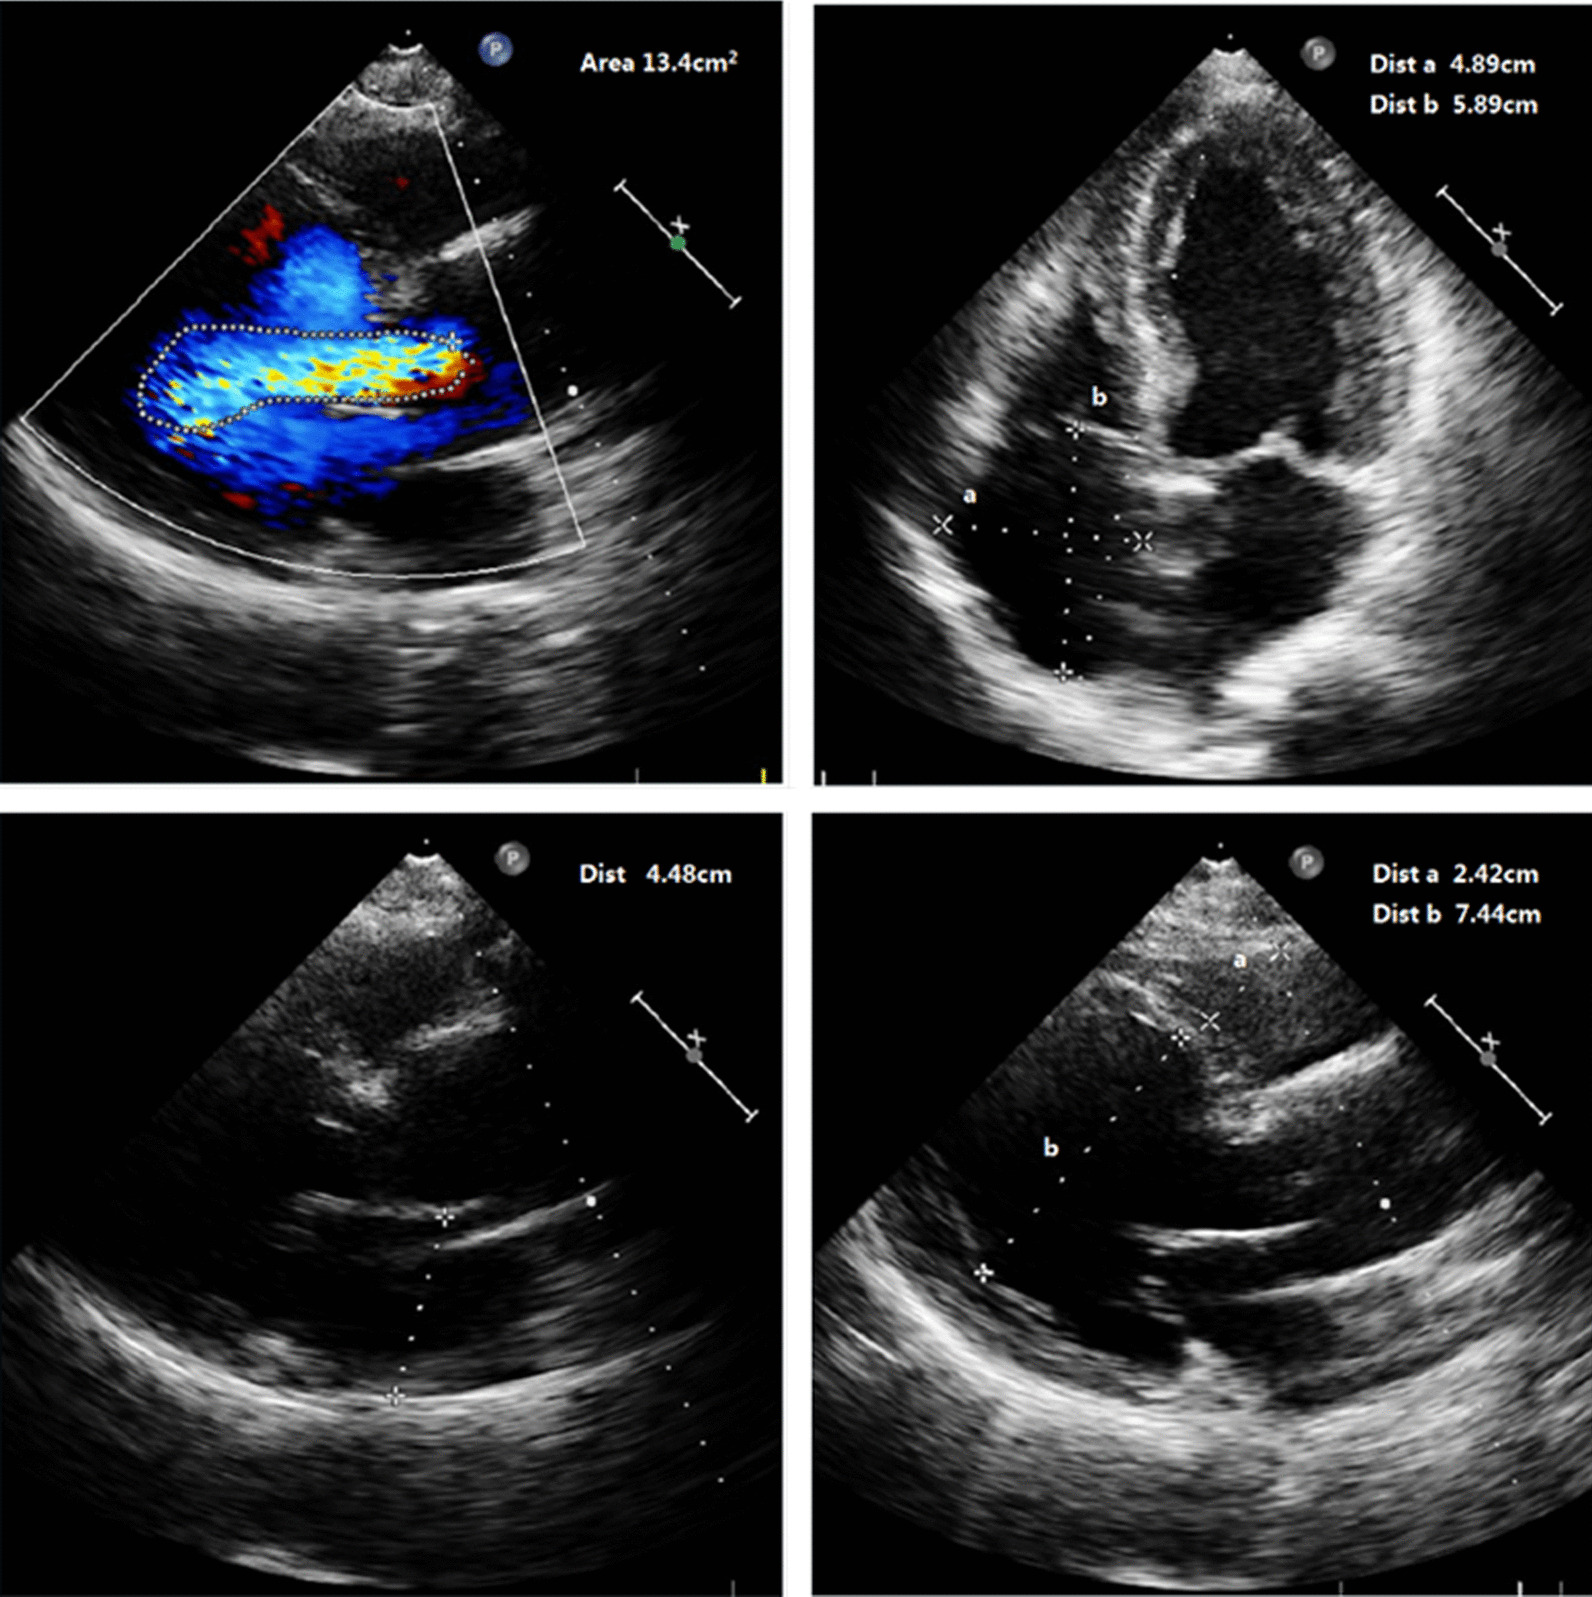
Preoperative echocardiography
